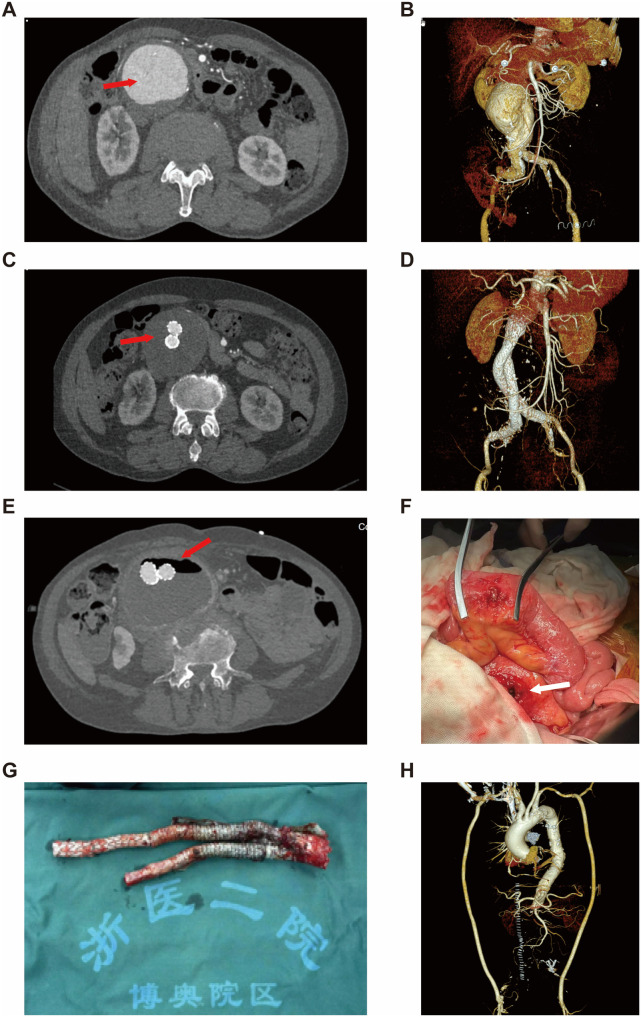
Imaging and intraoperative findings of the case report. (A) Preoperative CTA on September 12, 2022, demonstrating an infrarenal abdominal aortic aneurysm with a maximum diameter of approximately 77 mm (red arrow). (B) Three-dimensional (3D) reconstruction of the same preoperative CTA, clearly showing the overall morphology, tortuosity, and extent of the infrarenal abdominal aortic aneurysm. (C) Follow-up CTA on January 28, 2023, showing the stent graft in place within the abdominal aorta and bilateral common iliac arteries, with a circumferential low-attenuation rim around the graft aneurysmal dilatation of the sac with a maximum diameter of approximately 91 mm (red arrow). (D) 3D reconstruction of the same follow-up CTA corresponding to panel C. (E) CTA on January 15, 2025, demonstrating postoperative changes following EVAR with covered stent graft and iliac artery balloon angioplasty, with multiple gas shadows visible within the original aneurysmal sac (red arrow). (F) Intraoperative photograph during exploratory laparotomy, revealing an ulcerative lesion of the ileum approximately 260 cm distal to the ligament of Treitz, adherent to the abdominal aortic lesion (white arrow). (G) Photograph of the explanted endovascular stent graft removed during reoperation. (H) 3D reconstruction of CTA performed one week post-operatively.
